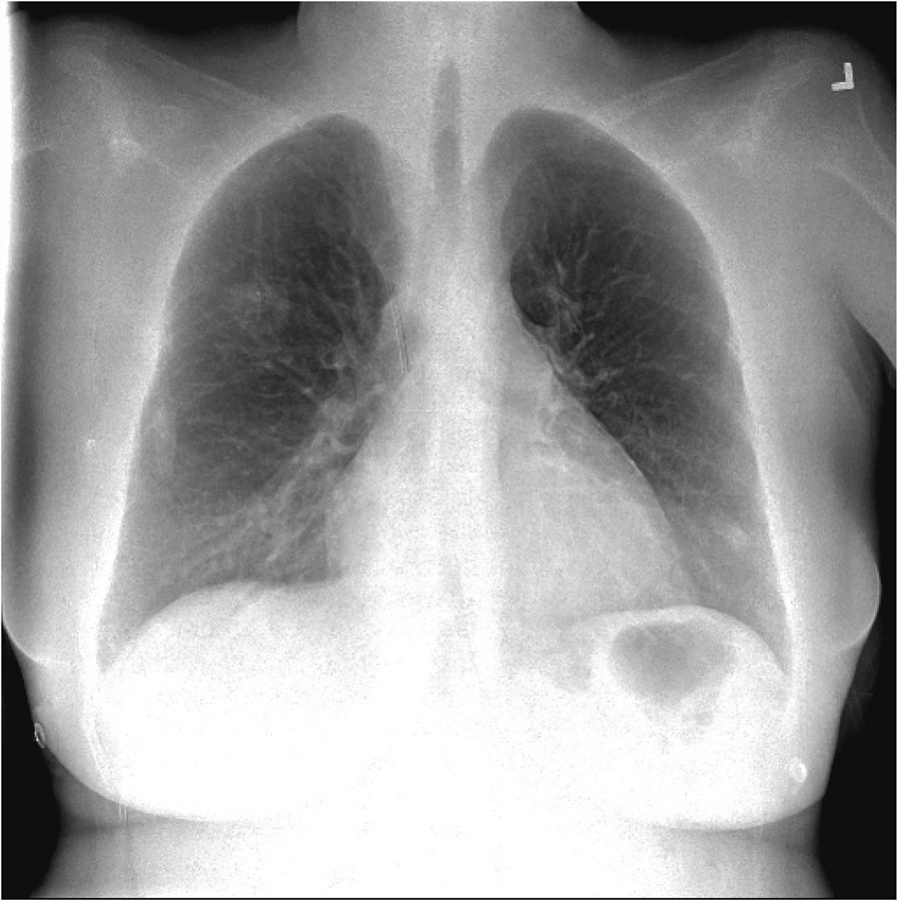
An anteroposterior chest radiograph demonstrating hazy bibasilar interstitial infiltrates. A port is noted as well in the right upper chest with catheter ending at the cavoatrial junction
